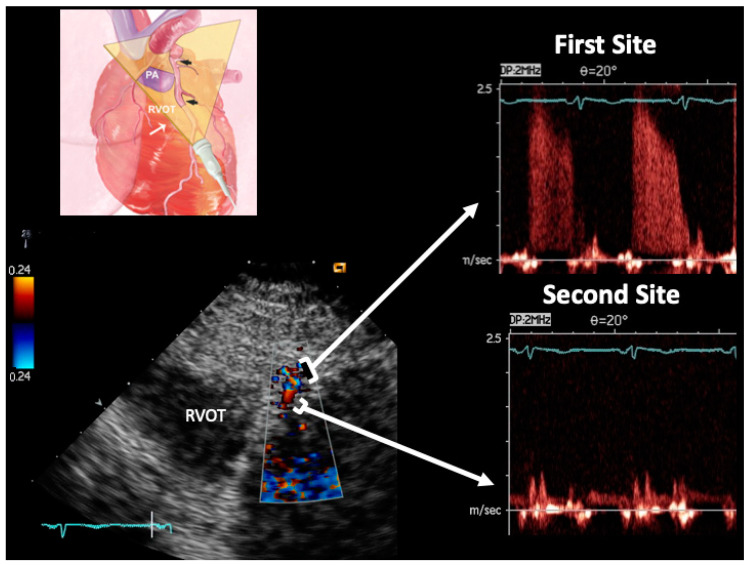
Example of PW Doppler sampling guided by color flow imaging performed at a stenotic site and a reference site in a case of significant stenosis. Same arrangement as Figure 1. In this case, the LAD segment involved is in the mid LAD (see the different plane orientation); the blood flow velocities are much higher at the stenosis site since AsF = 1050%; the %CSA calculated by using TVIs gave a %CSA = 94% (a corrected equation was adopted since the AsF value was >120%). Legends are the same as those in Figure 1.
